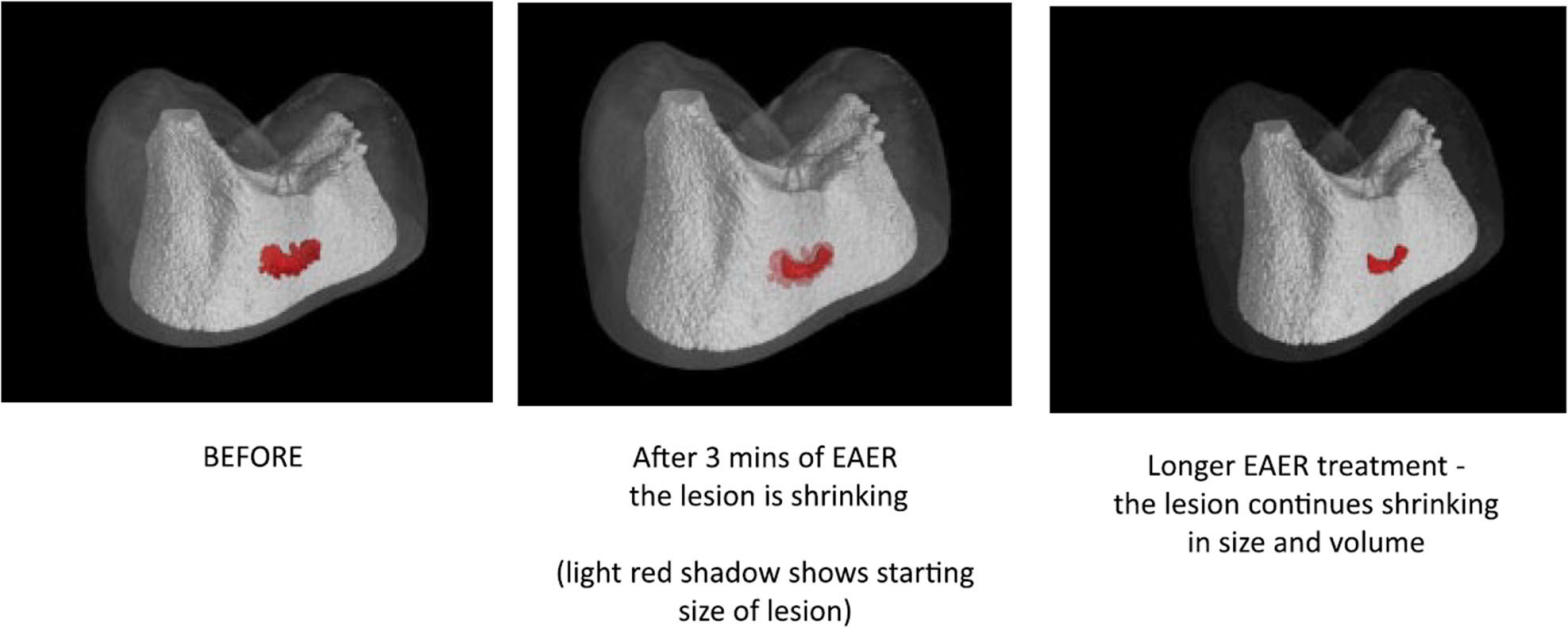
Three-dimensional X-ray mercury cadmium telluride images of an EAER-treated lesion. EAER-treated lesion reducing in volume and size. The red colouring represents the area of the enamel lesion that had a calculated mineral density ≥5% lower than the surrounding ‘healthy’ enamel [82]
